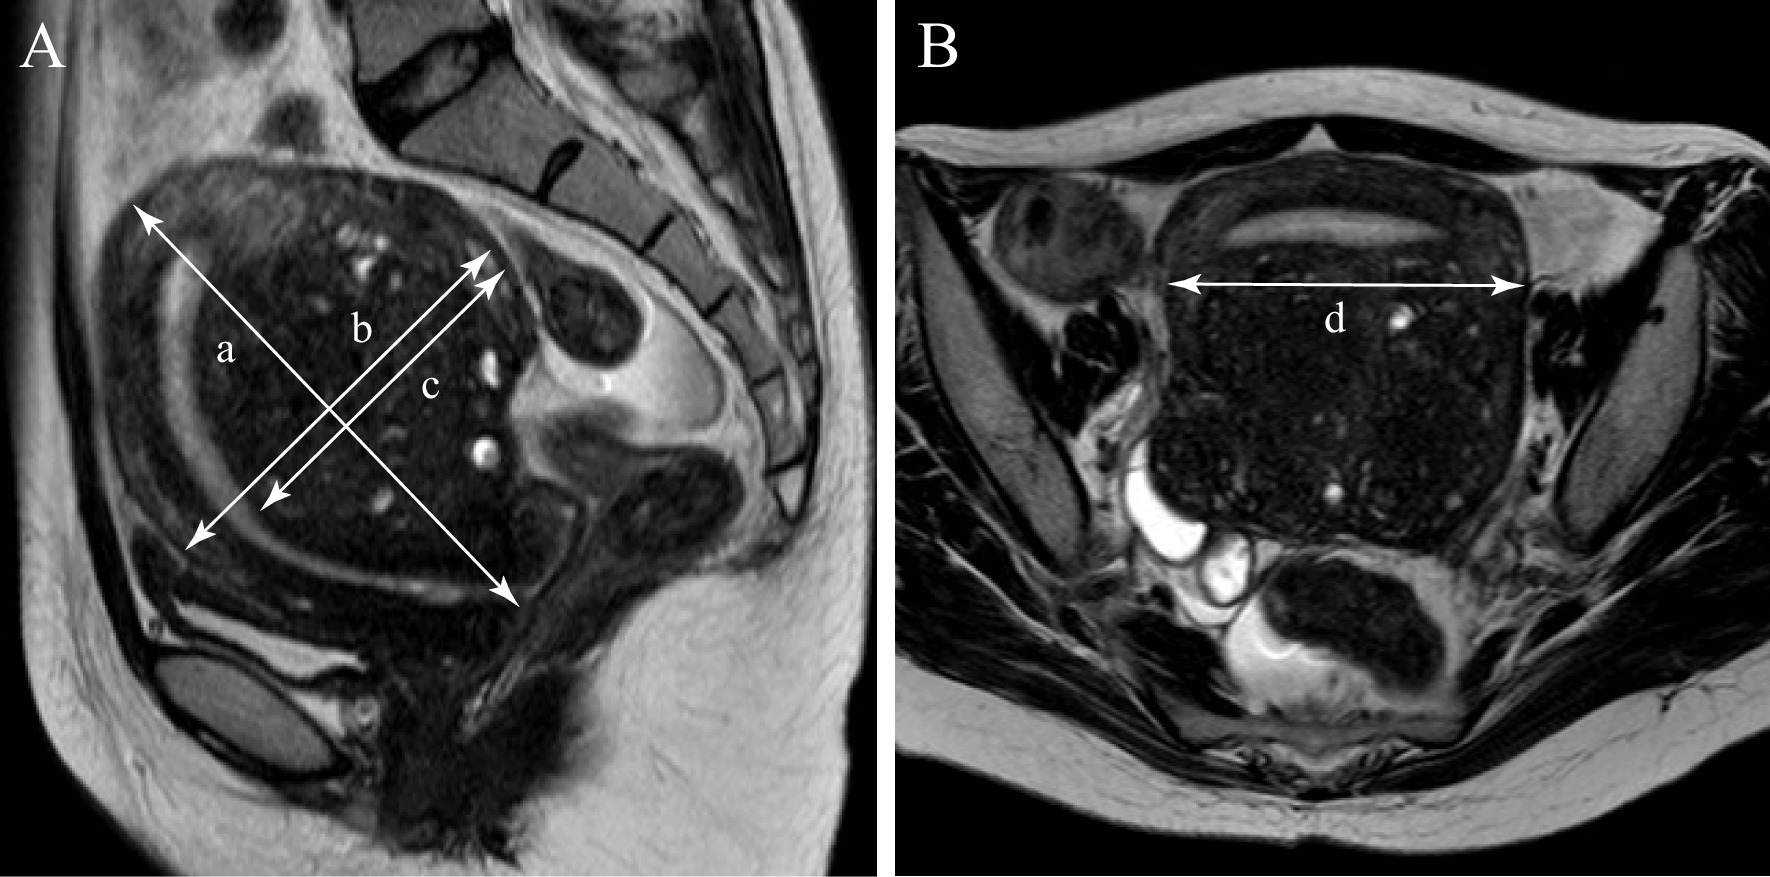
Measurement of uterine size. T2-Weighted Image (T2WI) of MRI. A We used the sagittal T2WI of the uterus to measure (a); the uterine long axis diameter, (b); the uterine short axis diameter and (c); the muscle layer thickness. B We used the axial T2WI of the uterus to measure (d); the uterine transverse diameter
